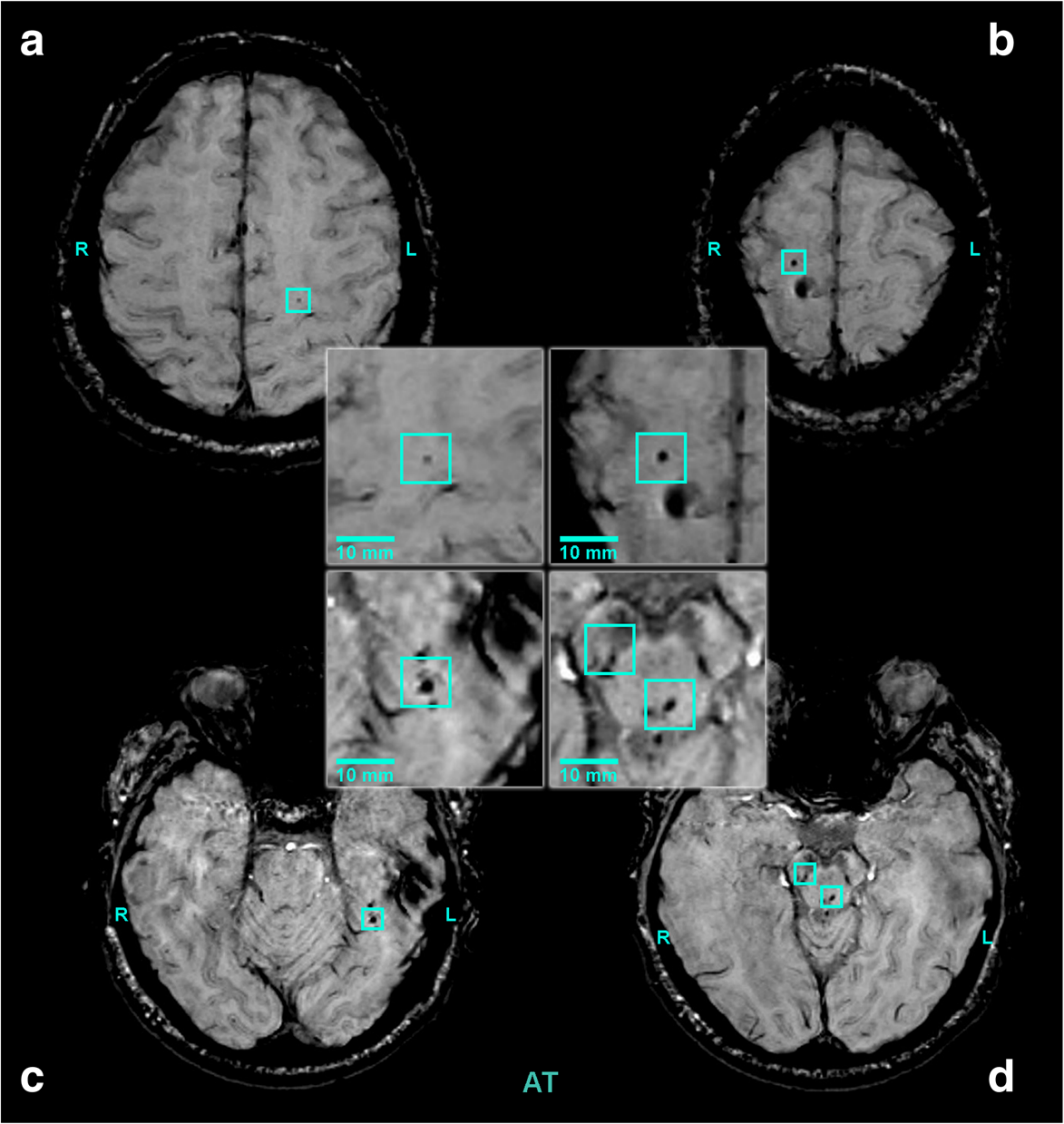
Axial susceptibility-weighted (SWI) magnetic resonance images (3 T) of an elderly patient (case 2) with mild traumatic brain injury (AT) (60-year-old, male). Cerebral microbleeds (CMBs) are highlighted by the blue boxes: A, left corona radiate; B, right corona radiate; C, left parahippocampal gyrus; D, crus cerebri, medial longitudinal fasciculus
